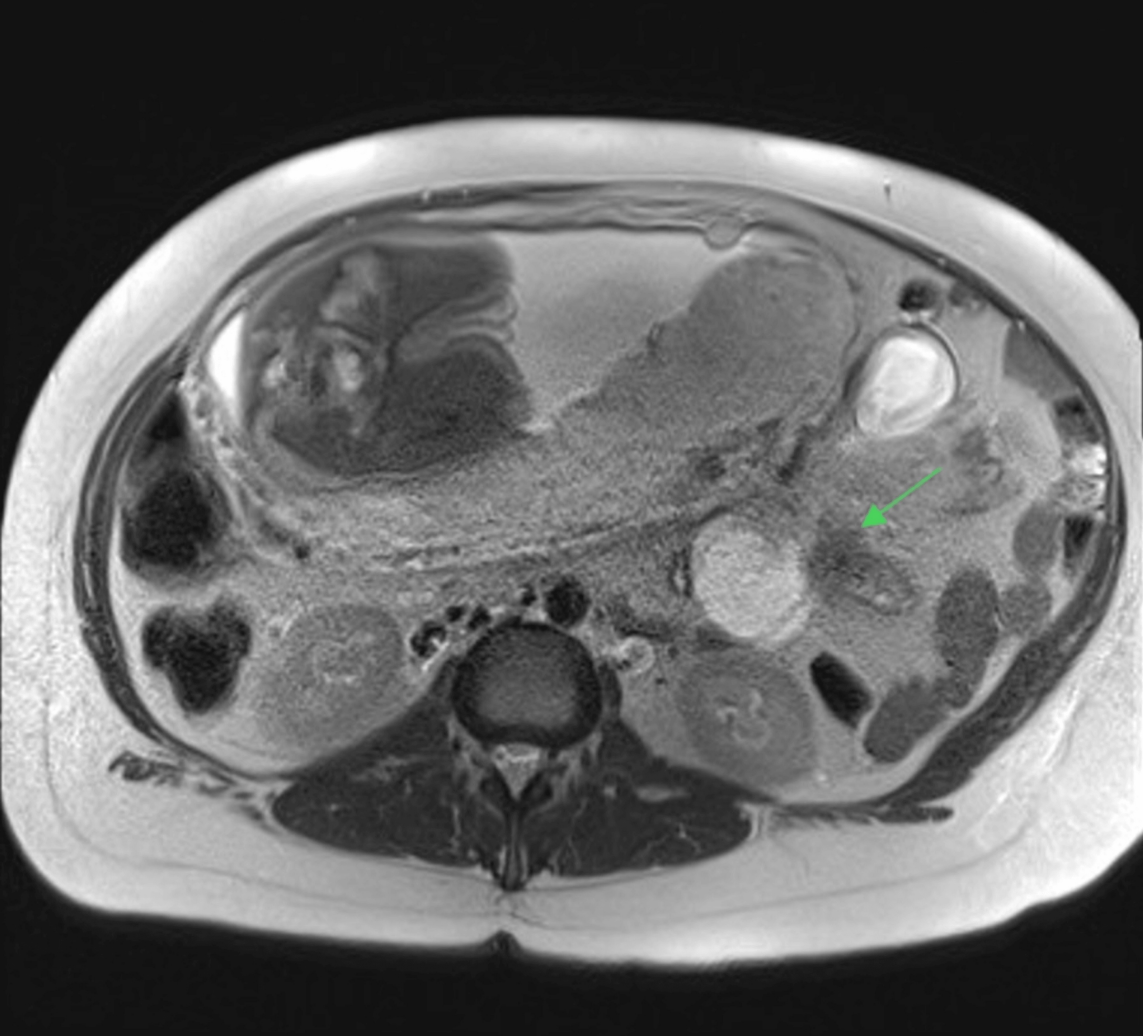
MRI T2 axial plane demonstrating internal herniation of small bowel through Petersen's space
